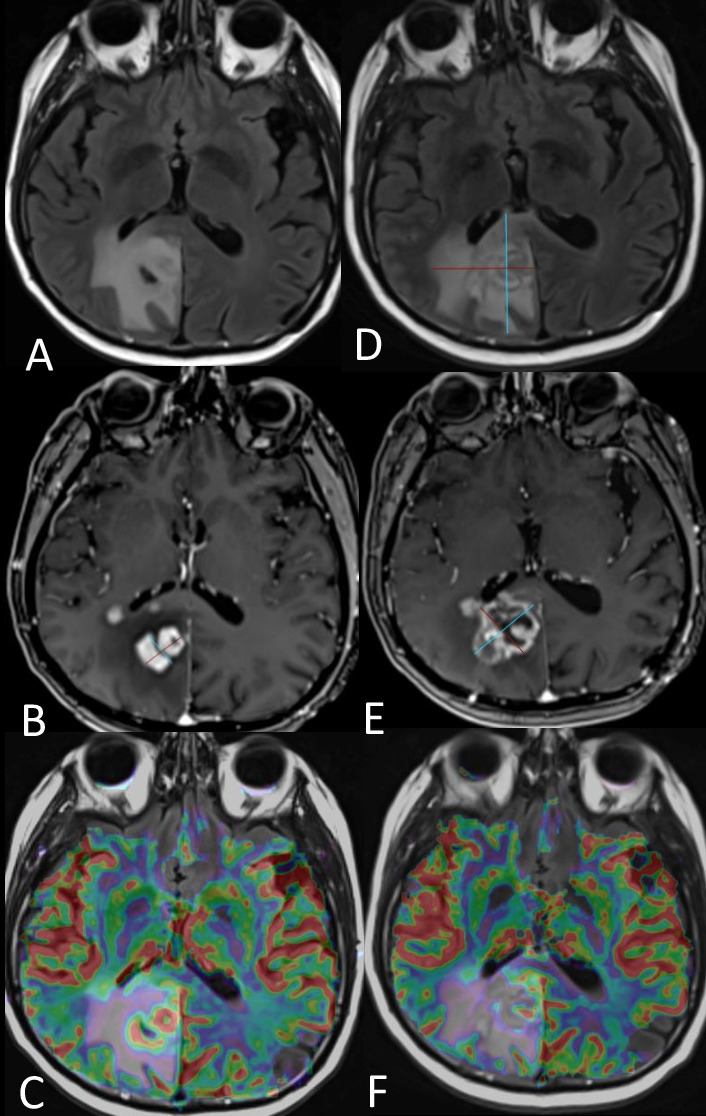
Patient with right occipital glioblastoma underwent diagnostic biopsy and concurrent adjuvant chemoradiation with temozolomide. 6 months after the baseline MRI (A–C), which showed well-circumscribed oedema (arrow in A) with rather solid enhancing (arrow in B) tumour and strong perfusion features in the DSC-derived rCBV map (arrow in C), there is further increase in the oedematous area (arrowhead in D) with expansile partly necrotic tumour mass (arrowhead in E). According to the mRANO criteria, the lesion is classified as preliminary progressive disease but the obvious decrease in the tumour vascularity in the rCBV map (arrowhead in F) demonstrates clearly the pseudoprogression effect. The enhancing lesion was stable in the next MRI follow-up and started resolving 3 months later. DSC, dynamic susceptibility contrast; RANO, Response Assessment in Neuro-Oncology; rCBV, relative cerebral blood volume.
